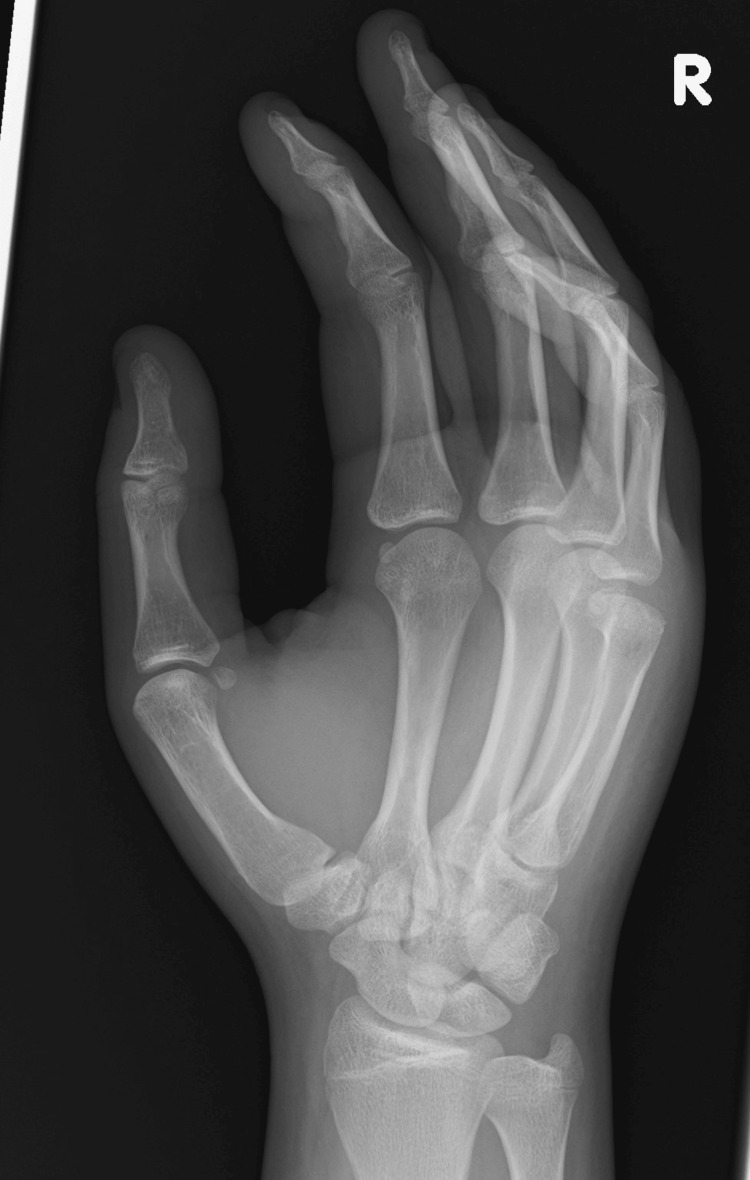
The patient's oblique radiograph taken on presentation to the emergency department.
